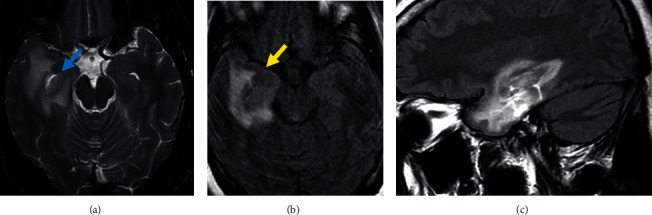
Axial T2-weighted (a), axial FLAIR (b), and sagittal FLAIR (c) views of MRI brain showing CNS relapse. Images show T2 isointense-hypointense signal in right inferior temporal lobe with peripheral edema causing expansion of the uncus (yellow arrow) without mass effect on temporal horn of right lateral ventricle (blue arrow).
